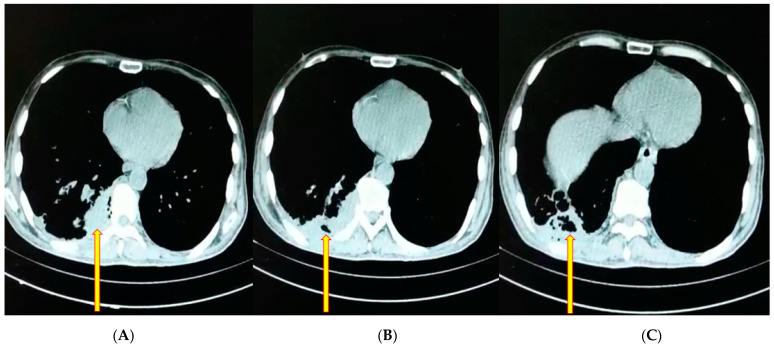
Computed tomography of the chest. (A) Axial computed tomography (CT) in a 51-year-old man showing right lower lobe nodules and opacities, bilaterally. (B,C) CT images showing necrotizing pneumonia with air picture within the pulmonary infiltrate, indicated by arrows. These features are relevant to a necrotic lung fragment in the angio-invasive form of pulmonary aspergillosis and co-morbid COVID-19 (CAPA), pulmonary tuberculosis, and type 2 diabetes mellitus with moderate diabetic ketoacidosis.
